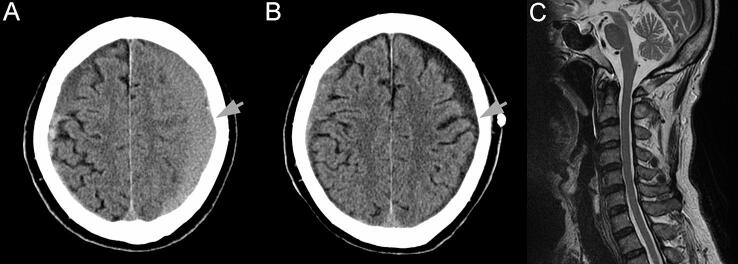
Head computed tomography (CT) and cervical spine magnetic resonance imaging (MRI). (A, B) Axial CT of the head before (A) and 7 days after (B) surgery. Note the absence of recurrence of the subdural hematoma and the absence of any apparent lesions in the brain on the left side (arrows). (C) Sagittal MRI of the cervical spine 3 days after symptom onset. Neither spinal cord compression nor intra-axial lesions were observed.
